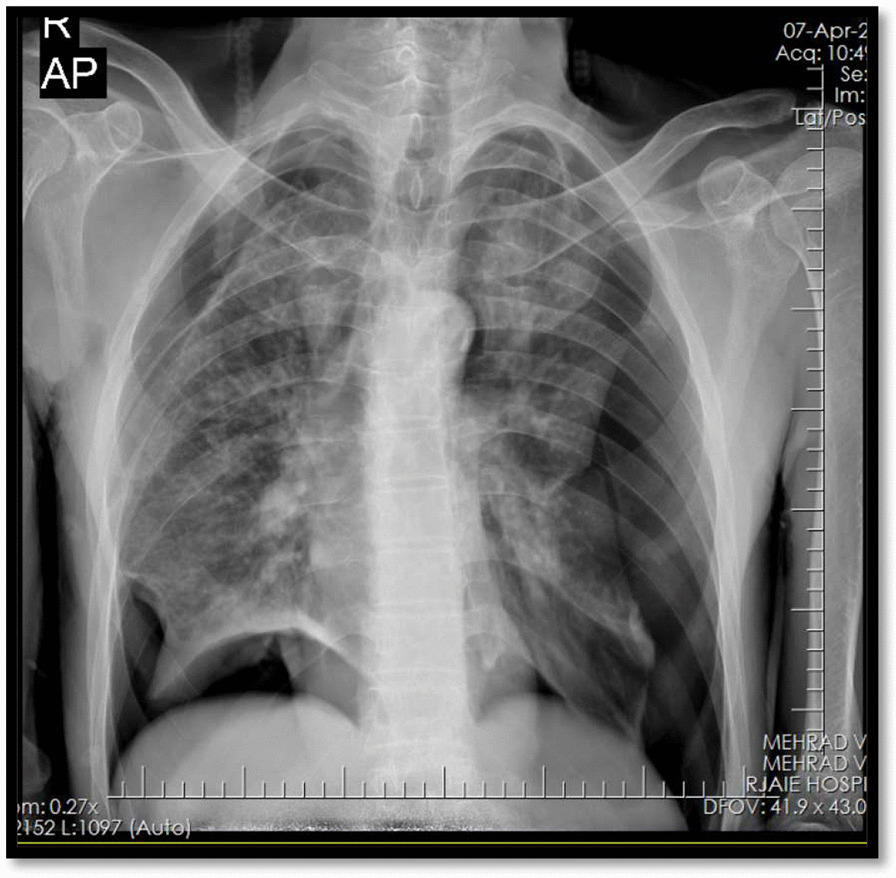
Chest radiography (1st admission), bilateral primary spontaneous pneumothorax
